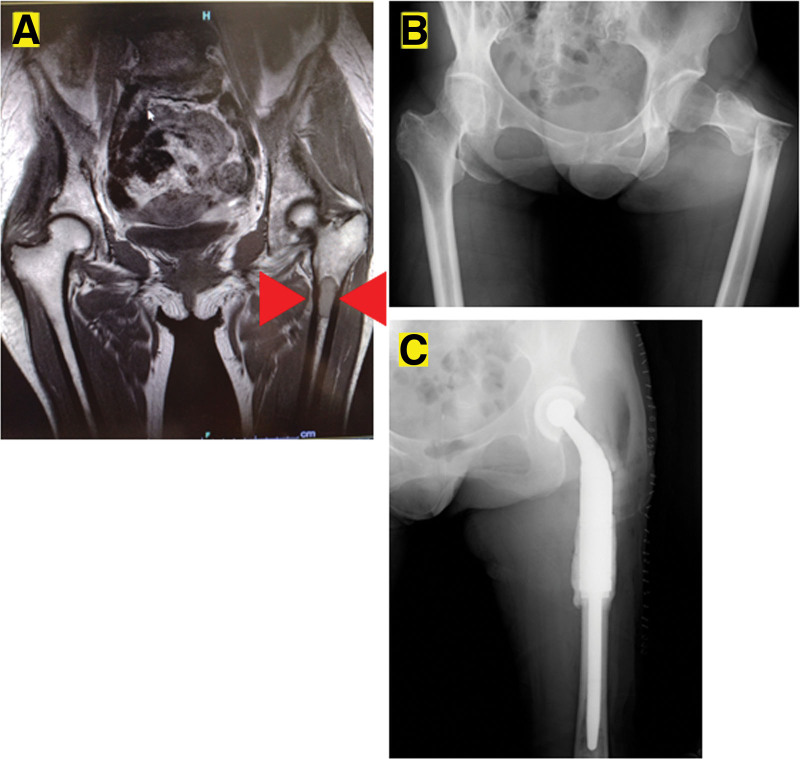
Magnetic resonance image of the hip (A). Metastasis region of the left femur is observed (A). Radiograph of the hip (B). The pathological fracture is observed (B). Radiograph of the left femur after surgical treatment with endoprosthesis (C).
